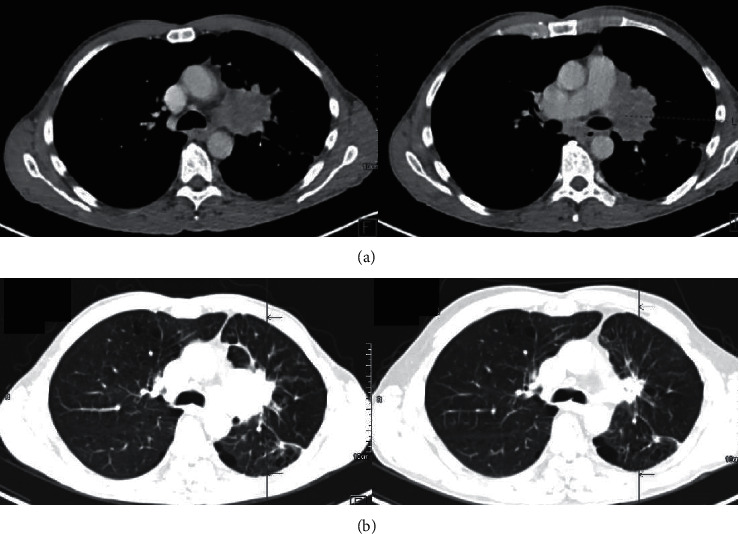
CT scans showing (a) left upper lobe SCC at diagnosis and (b) a partial response to chemoradiotherapy at 4 weeks.
